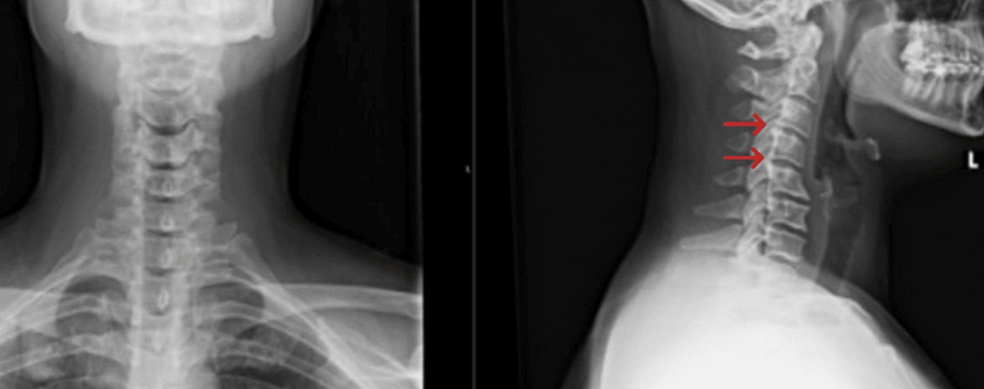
Cervical spine radiographs demonstrating straightening of the normal cervical lordotic curvature, multilevel degenerative changes with prominent anterior spurring, and notable foraminal narrowing at C3-C4 and C4-C5 (red arrows) L: left.
